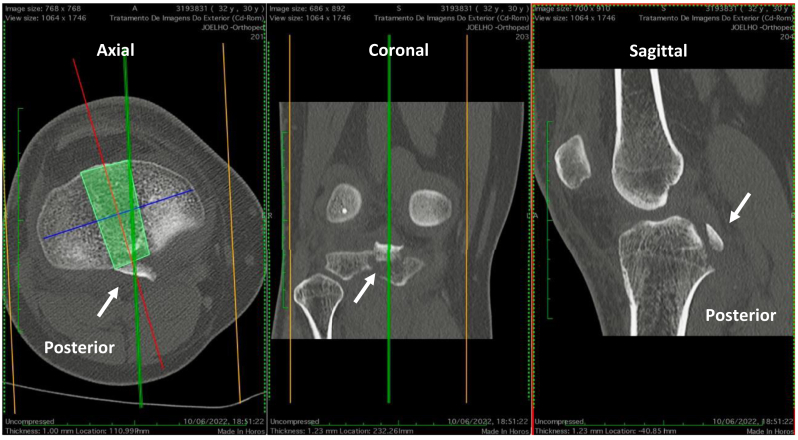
Computed tomography scan demonstrating the posterior cruciate ligament tibial avulsion fracture, including its size, grade of comminution, and the amount of displacement, an integral part of the preoperative imaging studies to plan the surgery.
